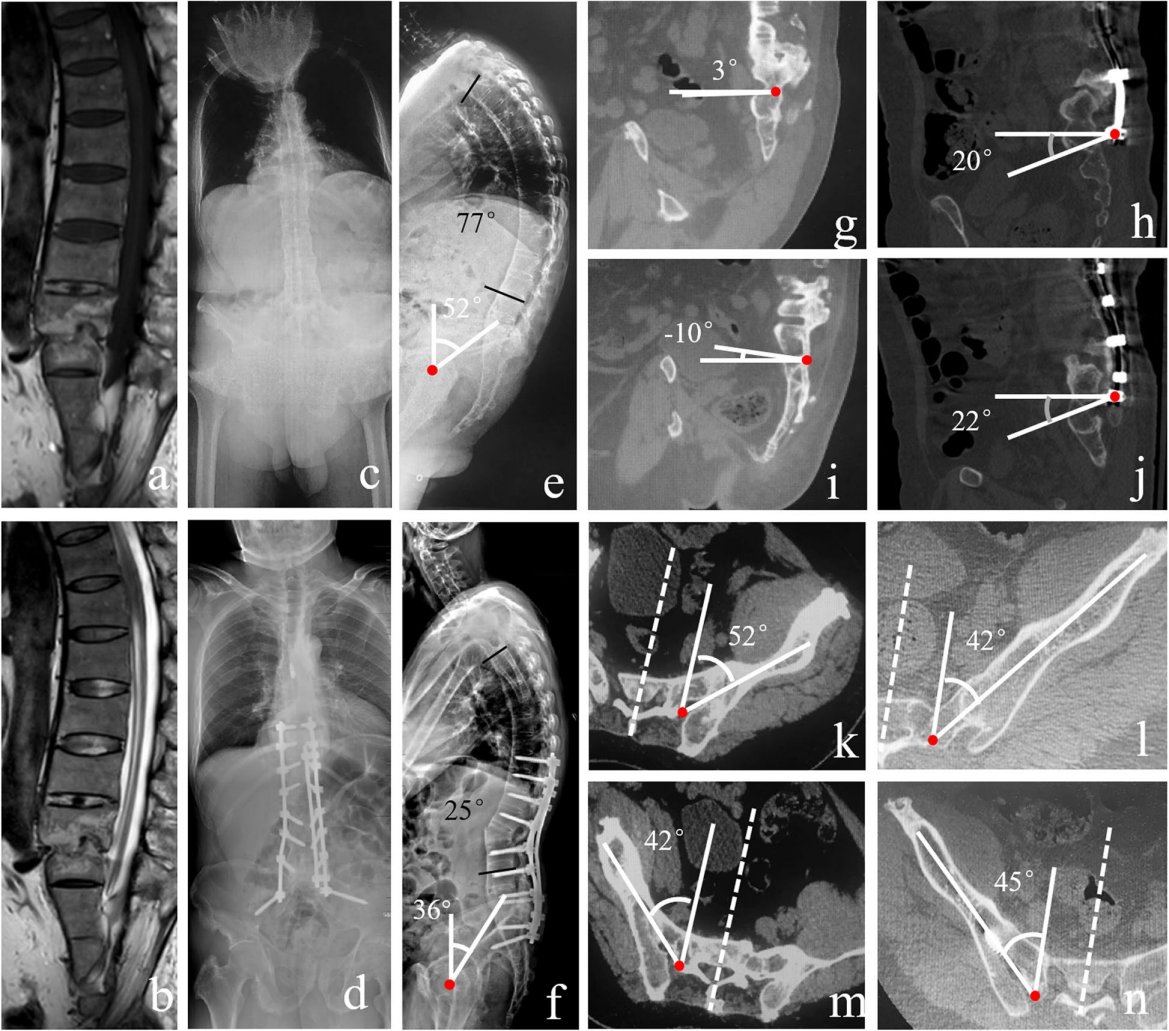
A 55-year-old male AS patient with L5/S1 pseudarthrosis and thoracolumbar kyphosis underwent L2 PSO combined with bilateral S2AI screw fixation a-b A L5/S1 pseudarthrosis was shown on the MR images (a: T1-weighted; b: T2-weighted). c-d Coronal view. e-f On the standing lateral radiograph, the GK (77° vs 25°) and PT (52° vs 36°) of the patient were improved after surgery. g-j The lateral view of S2AI trajectory on the CT imaging before and after surgery on both sides. k-n The transverse view of S2AI trajectory on CT imaging
